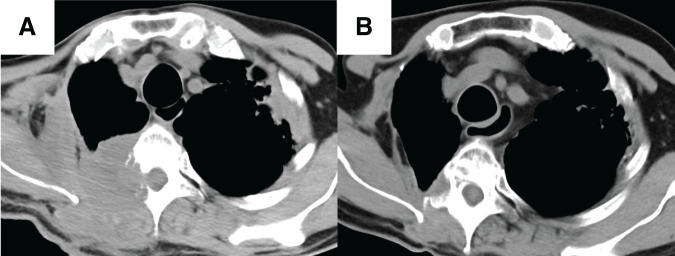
CT findings of a local recurrence after surgery. We found a local recurrence in the CW that had invaded the 3rd thoracic vertebra (A). The recurrent lesion responded to treatment and a complete response was achieved (B).
